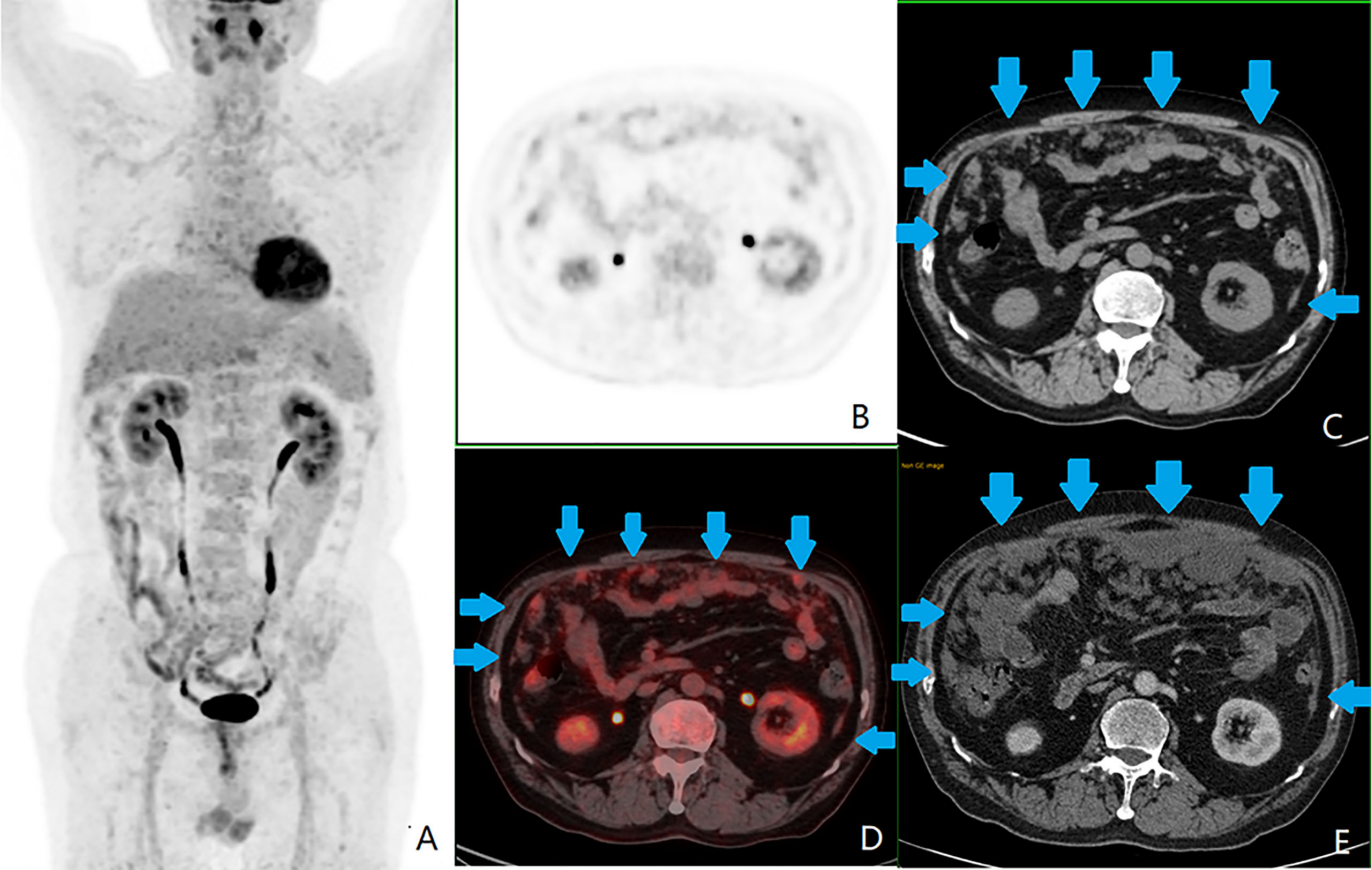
A patient with colorectal peritoneal carcinomatosis (CRPC), male, 68 years old, with mucinous adenocarcinoma of the appendix. (A) The positron emission tomography (PET) 3D-MIP image; (B) PET cross-sectional image; (C, E) computed tomography (CT) cross-sectional image; (D) PET/CT fusion cross-sectional image; (A–D) the first ^18^F-fluorodeoxyglucose PET/CT examination on October 11, 2016, which showed CRPC distributed in zones 0 ([lesion size] LS-2), 1 (LS-2), 2 (LS-2), 3 (LS-2), 4 (LS-2), 5 (LS-2), 6 (LS-2), 7 (LS-2), and 8 (LS-2), as shown by the blue arrow in images (C, D). This patient had a peritoneal cancer index (PCI) score of 18. The largest lesion was located in the umbilical region (zone 0), measuring approximately 3.9 cm, with a standardized uptake maximum value of 4.8 g/ml with a small amount of seroperitoneum. (E) Contrast-enhanced abdominal and pelvic CT examination on September 14, 2019, showed significant progression of CRPC, with a PCI score of 26.
